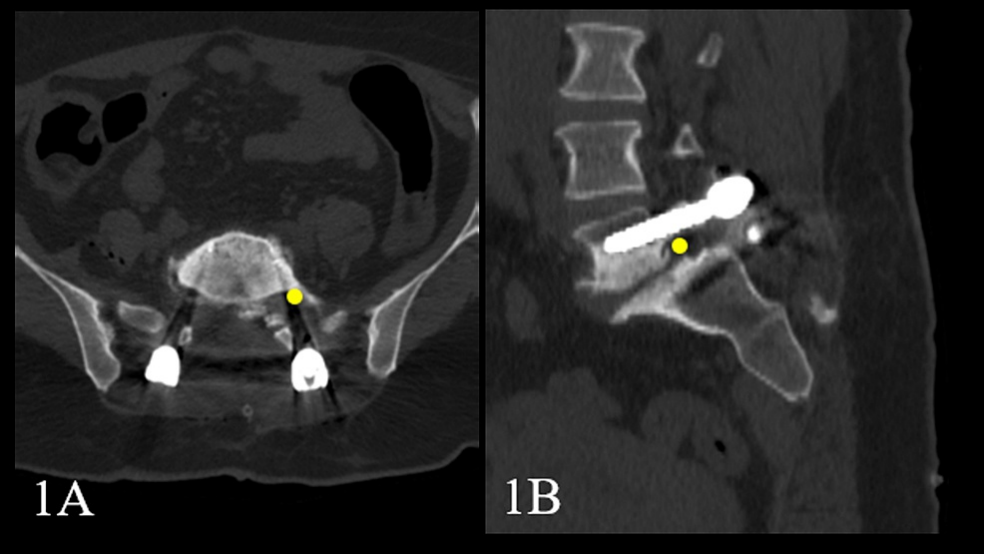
The lumbar 3D CT images demonstrate the target point where the needle tip is planned to be placed to perform L5 transforaminal nerve injection (yellow dot) in (A) axial and (B) in sagittal planes.
